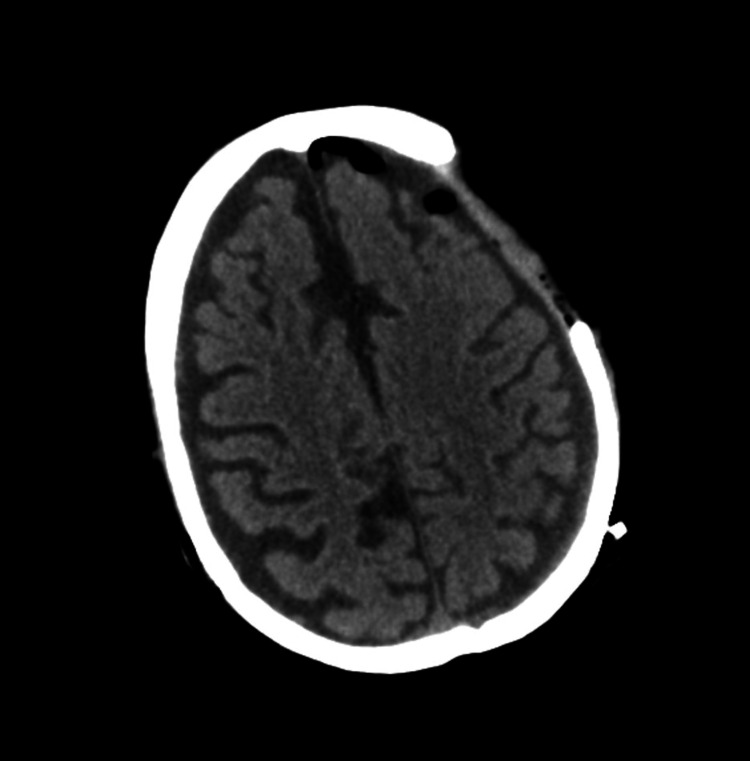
CT of the brain without contrast, post-craniectomy, showing a large area of removed bone and areas of pneumocephalus.
